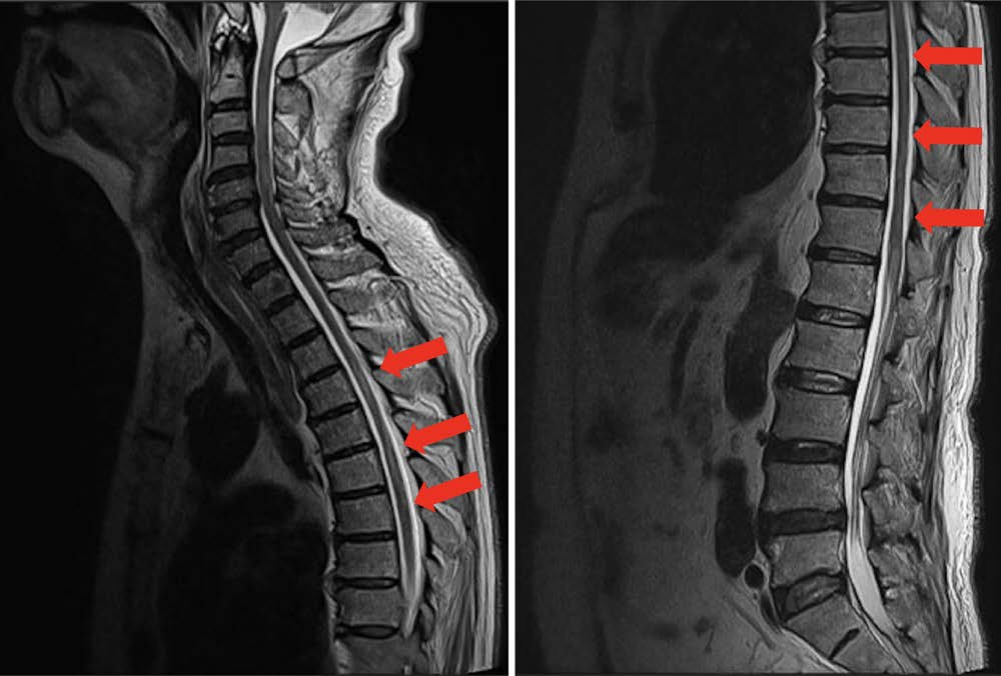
T2-weighted sagittal magnetic resonance image showing spinal cord infarction [red arrow].
